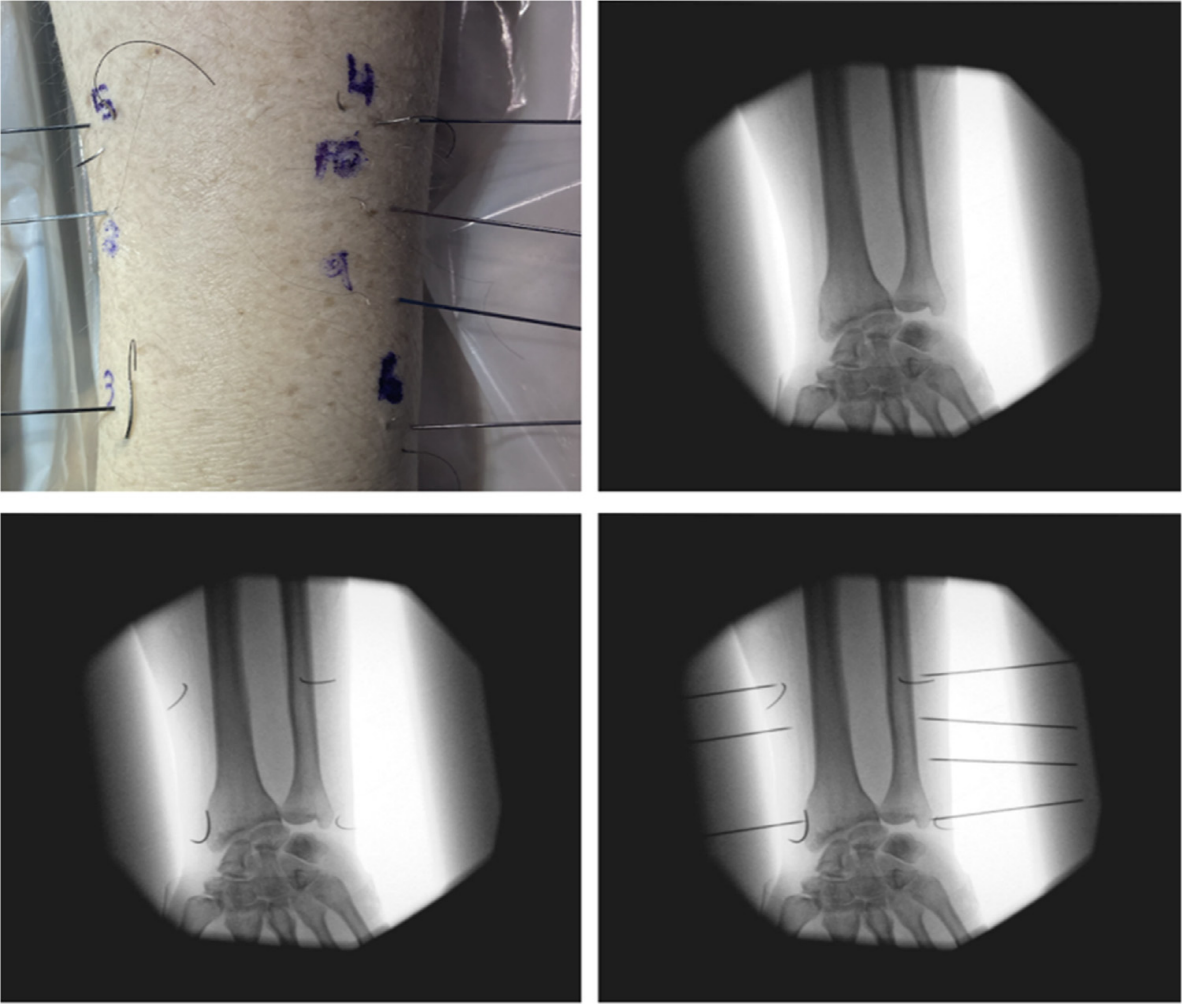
Distal Radius images/radiographs for needle identification.
